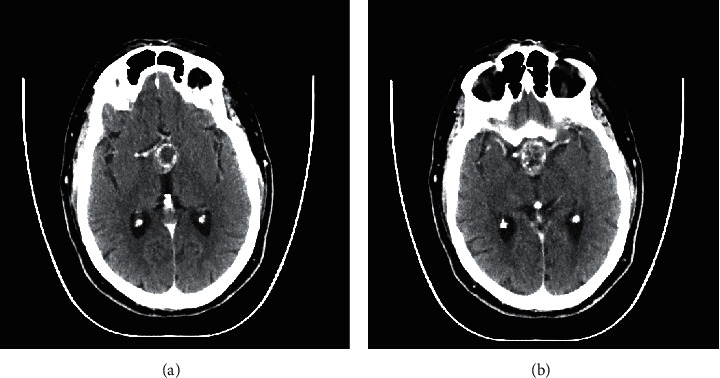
CT with contrast obtained by neurosurgery and otolaryngologist the day before surgery revealing a 2.7 cm × 2.3 cm × 1.9 cm suprasellar mass with peripheral calcifications. The mass impinges on the foramen of Monro and the anterior portion of the third ventricles.
